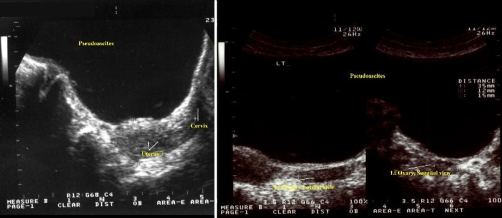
Transabdominal sonogram of (Left) the uterus and (Right) the left ovary of the patient.
